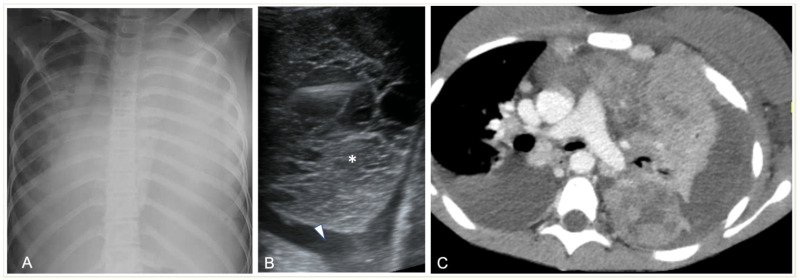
Imaging studies of a 14-year-old girl with respiratory distress, fever, and biopsy-proved non-Hodgkin Lymphoma. (A) Chest radiograph shows complete opacification of left hemithorax with mediastinal shift and some right lung opacities with pleural effusion. (B) LUS shows small left-sided pleural effusion (arrowhead) surrounding a lung mass (*) with multiple fluid-filled cavities. (C) Axial contrast-enhanced CT scan shows an inhomogeneously enhancing left lung mass with multiple necrotic areas, thoracic wall infiltration, bilateral pleural effusions, and enlarged mediastinal lymph nodes.
